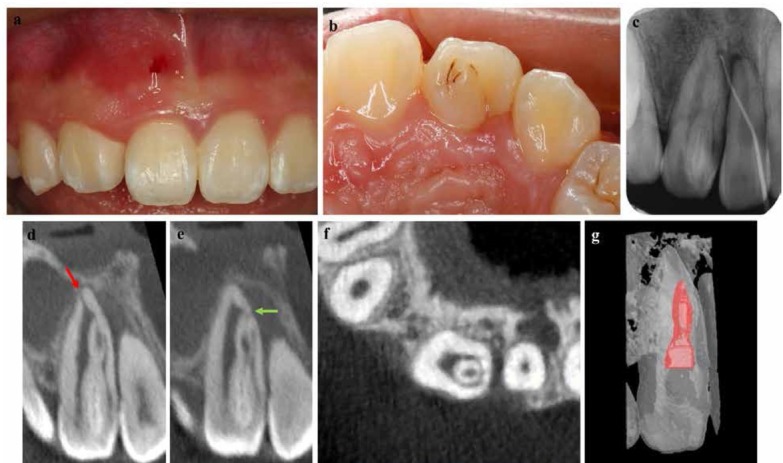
(a) Sinus tract with respect to tooth #8. (b) Unusual prominent lingual cusp and a deep lingual pit with respect tooth #7. (c) IOPA showing the sinus tract done by GP cone confirming the lesion on tooth#7. (d) CBCT image of the invaginated structure showing the main apical foramen (red arrow). (e)Axial view of CBCT image showing the invaginated with separate portal exit(green exit). (f) Axial view of CBCT showing the invaginated structure. (g) CBCT 3D reconstruction images demonstrating the invaginated structure.
